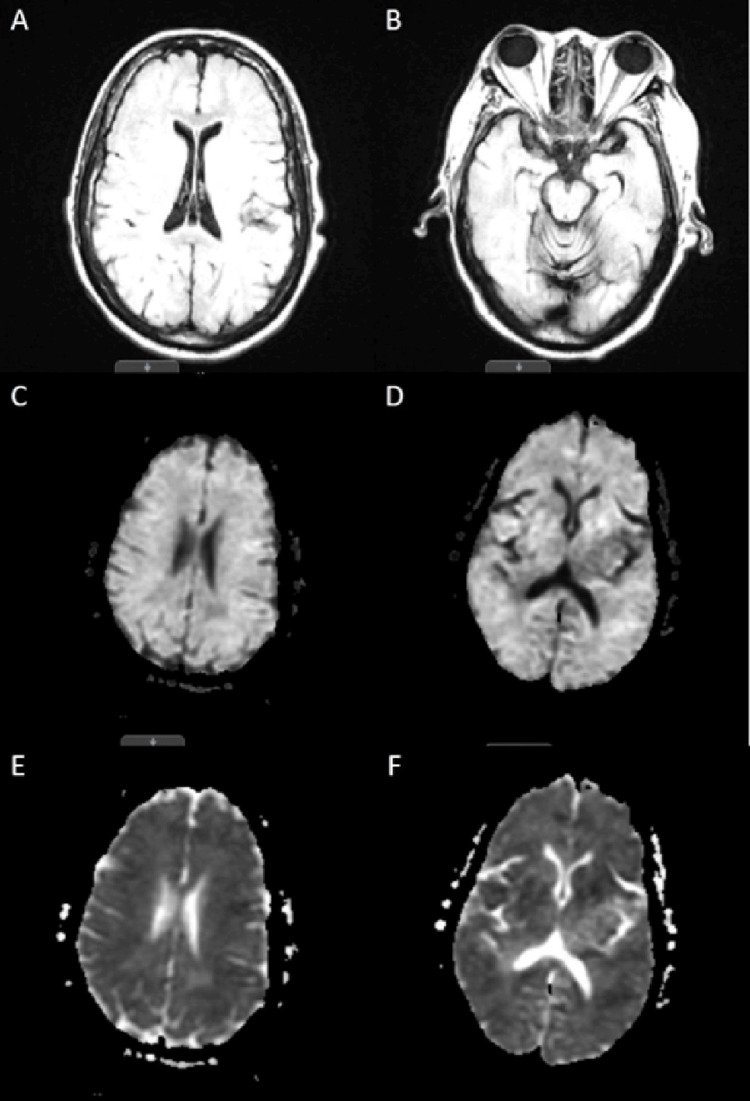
Non-contrast MRI of the head showing diffuse diffusion restriction, partial sulcal effacement, and mild diffuse cerebral edema. (A) Axial T2-weighted fluid-attenuated inversion recovery (FLAIR) image at the level of the frontal horn of the lateral ventricle. (B) Axial T2-weighted FLAIR image at the level of the midbrain. (C) Axial diffusion-weighted image (b1000) at the level of the lateral ventricles and inferior sagittal sinus. (D) Axial diffusion-weighted image (b1000) at the level of the atrium of the lateral ventricle. (E) Axial apparent diffusion coefficient (ADC) image at the level of the lateral ventricles and inferior sagittal sinus. (F) Axial ADC image at the level of the atrium of the lateral ventricle.
